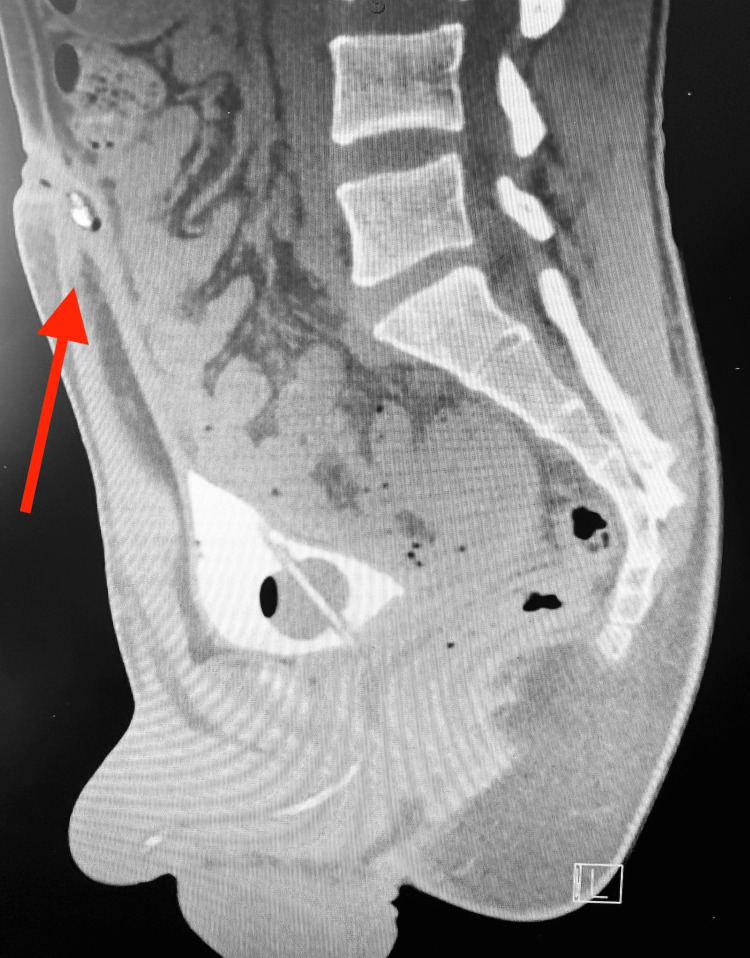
CT cystogram of the patient CT cystogram image showing a fibrous urachal remnant without evidence of a patent urachus or urachal cyst.
